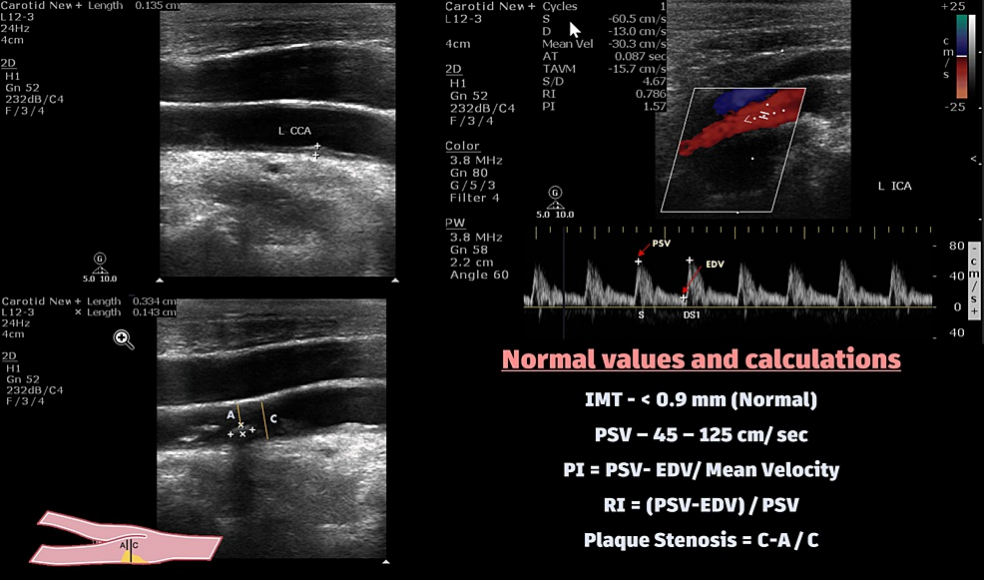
Assessment with carotid artery Doppler parameters and normal values IMT: intima-media thickness; PSV: peak systolic velocity; EDV: end diastolic volume; PI: Pulsatility Index; RI: Resistivity Index
